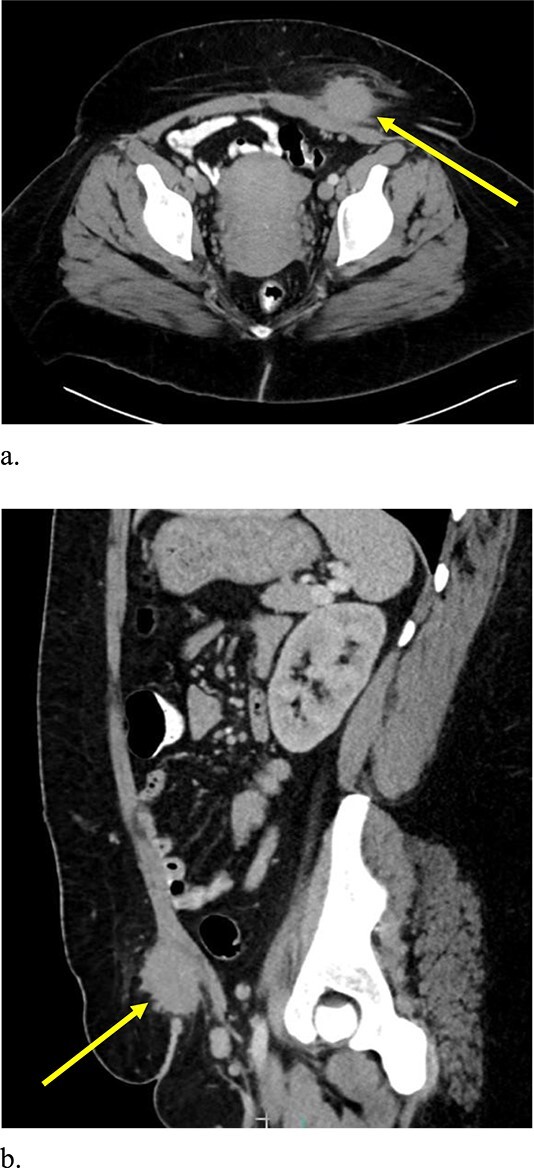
Computed tomography axial (a) and sagittal (b) views of a 4.2 × 4.1 × 3.7 cm enhancing, irregular mass on the abdominal wall (arrow) suggestive of AWE.
